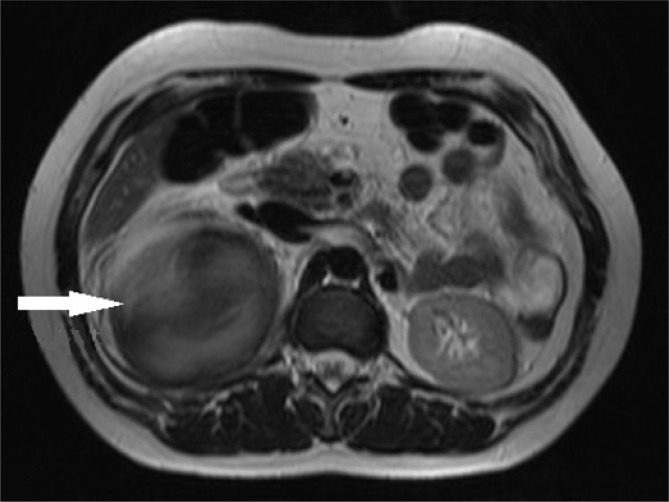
Axial abdominal magnetic resonance imaging cut of patient
